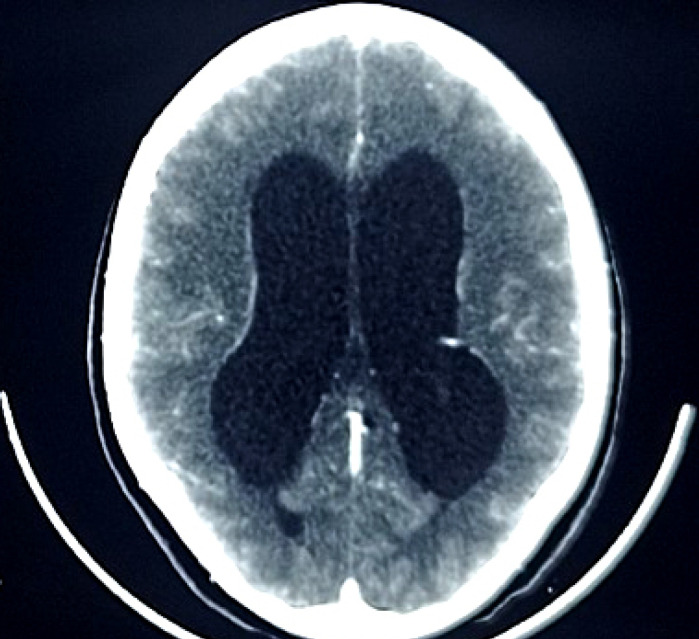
Tomodensitométrie cérébrale en coupe axiale après injection de produit de contraste: dilatation tétraventriculaire associée à des hypodensités rehaussées et diffuses de la substance blanche chez une patiente de 18 ans séronégative pour le VIH, atteinte de cryptococcose neuroméningée et tuberculose pulmonaire Cerebral CT scan in axial section after injection of contrast agent: tetraventricular dilation associated with enhanced and diffuse white matter hypodensity in an 18-year-old HIV-negative patient with neuromeningeal cryptococcosis and pulmonary tuberculosis
